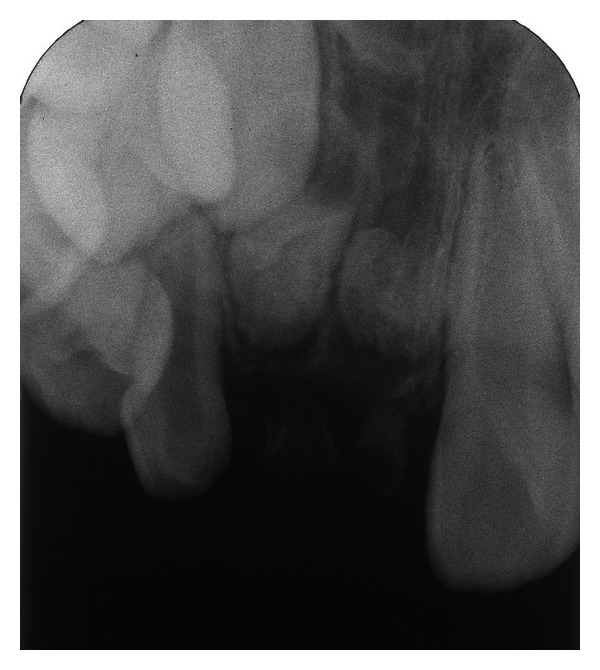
Periapical radiograph at age 9 years and 2 months (case 2). There is no evidence of eruption tendency.
